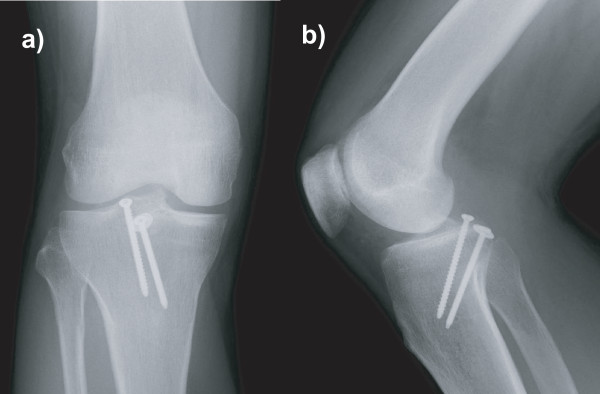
Radiographs (a and b) at the 3-month follow-up showing union of the bone fragments.
